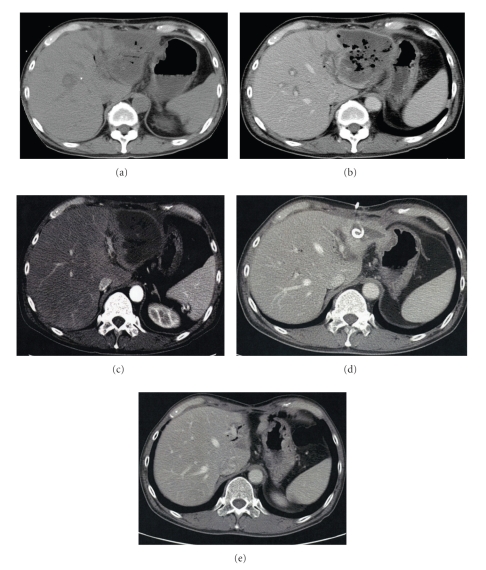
Representative images of CT performed after embolization. (a) 3 days after embolization. (b) 16th day after embolization. (c) 30th day after embolization. (d) 68th day after embolization. (e) 161 st day after embolization.
